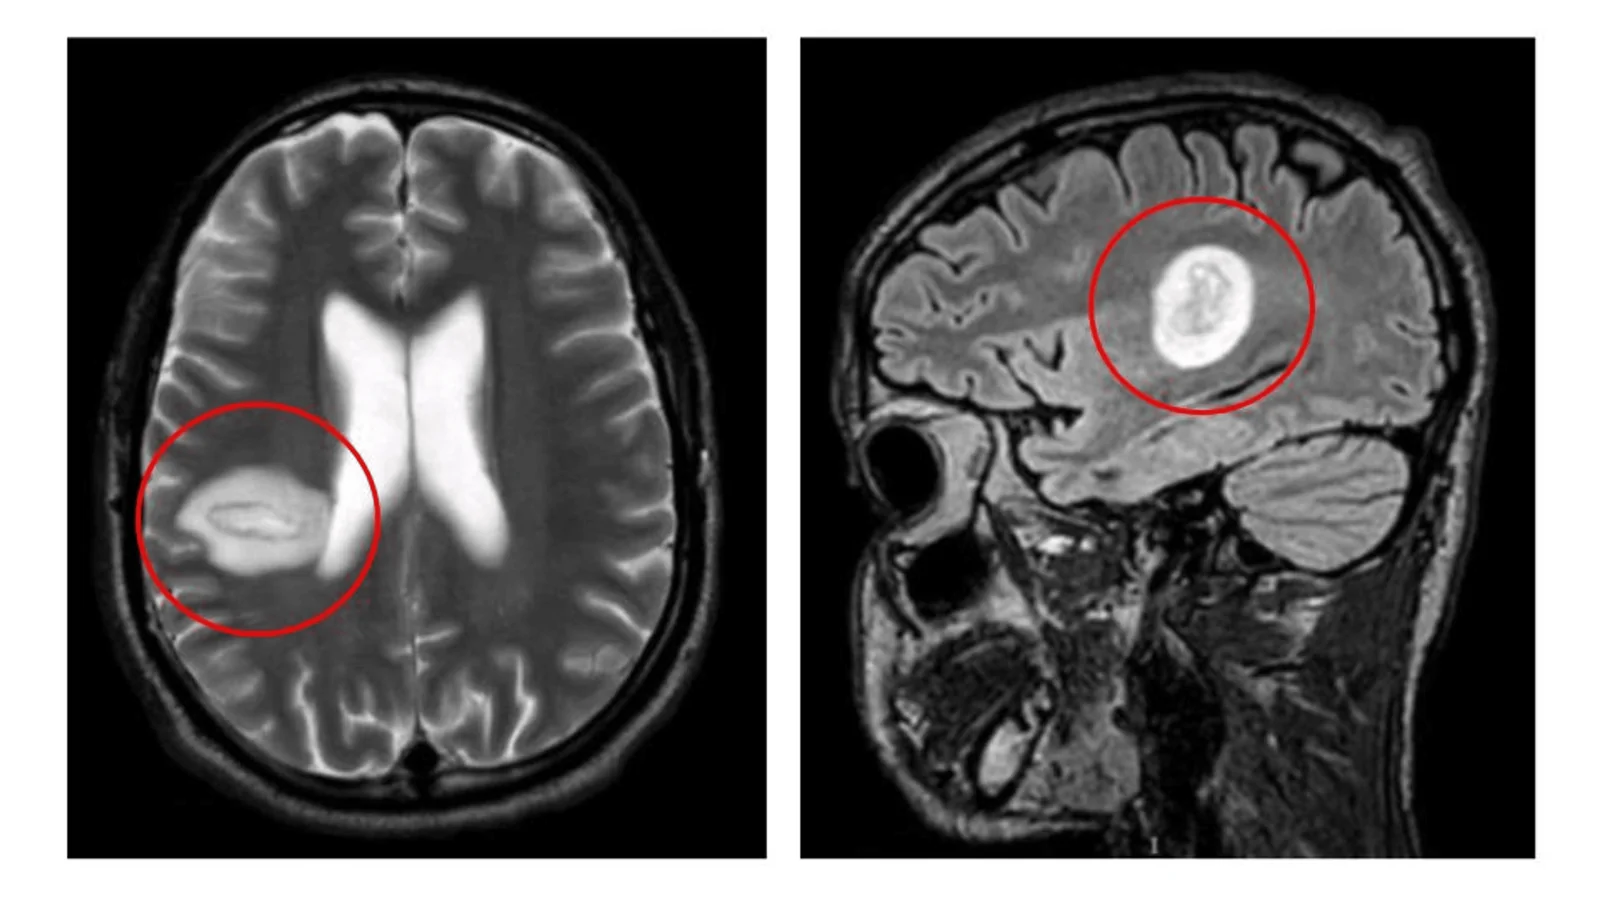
MRI brain with and without contrast showing a 2.8x1.2cm abscess in the right corona radiata
